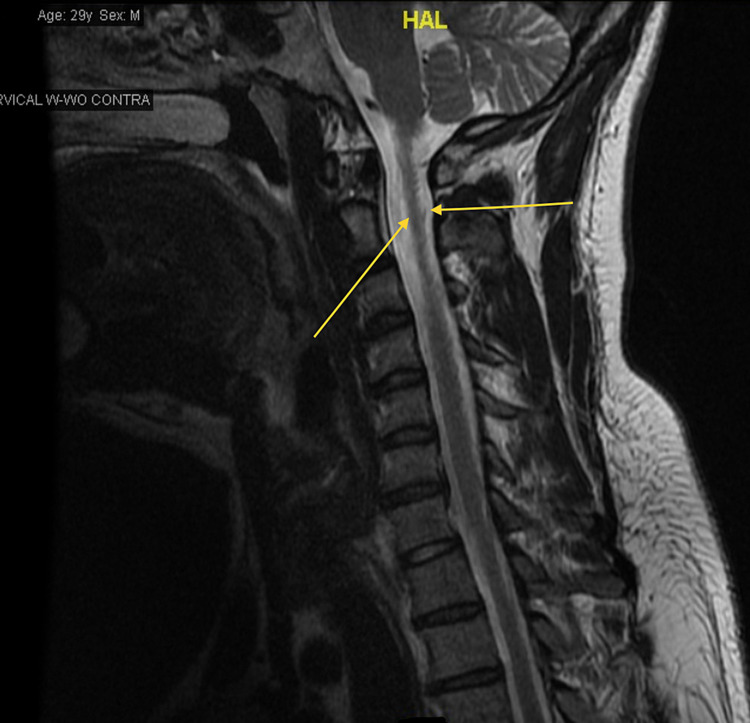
Multiplanar multi-sequence magnetic resonance imaging (MRI) of the cervical spine showing that the cervical cord demonstrated symmetric posterior medial signal abnormalities in a pattern consistent with vitamin B12 deficiency (yellow arrows). No other pathologic enhancement was seen within the cervical cord, meninges, or vertebral bodies.
